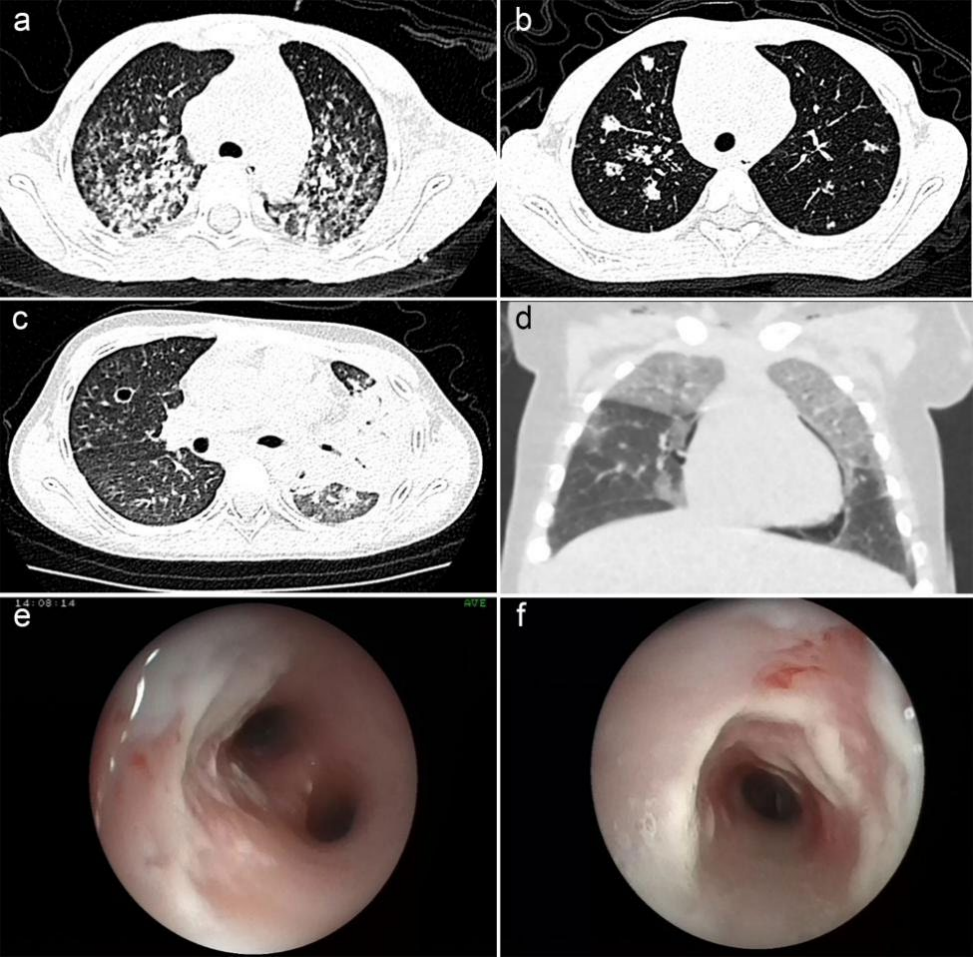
High-resolution computed tomography and tracheoscopic images show: (a) interstitial infiltration in both lungs, (b) distribution of multiple masses in the distal bronchus, (c) large consolidation of the left lung lobe with the pulmonary cavity, (d) mediastinal emphysema, (e, f) mucosal edema and erosion, and a large number of white adhesions in the intima of the trachea
